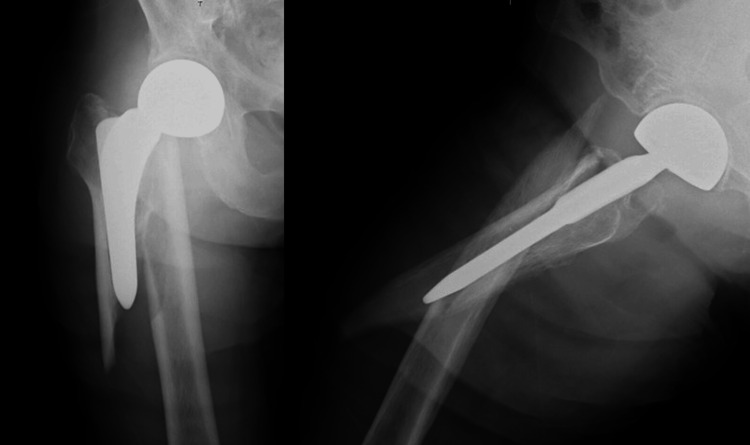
Preoperative radiographs of case 1 Preoperative radiographs of case 1 showed PFF of Vancouver classification Type B1. PFF: periprosthetic femoral fracture.
